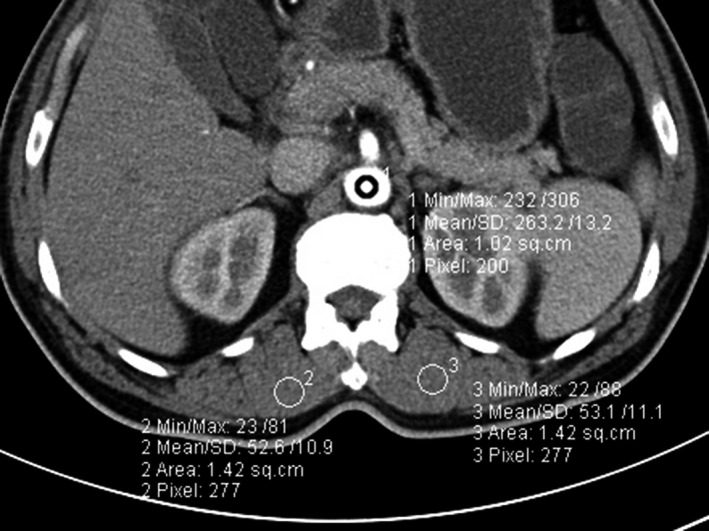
The contrast noise ratio (CNR) of the image at each stage via multislice CT enterography. CNR = (CT _blood vessels_ − CT_muscle_)/SD_muscle_.
